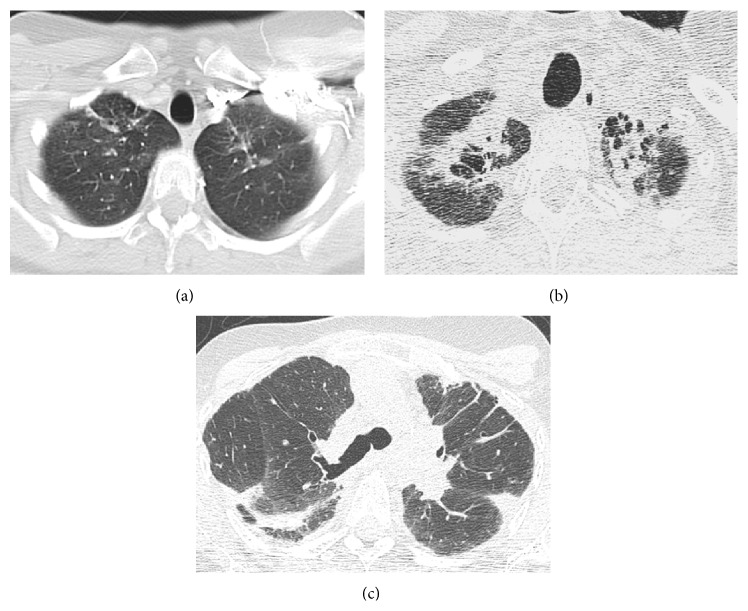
Case  2. (a) Axial HRCT scan shows very discrete lineal opacities in both lung apices. (b) Axial HRCT scan at the same level compared to (a) two years and two months later shows a loss of volume and prominent parenchymal fibrotic infiltrates. (c) Axial HRCT image at the level of carina reveals lung architectural distortion, as well as the presence of pleural thickening and subpleural parenchymal opacities.
